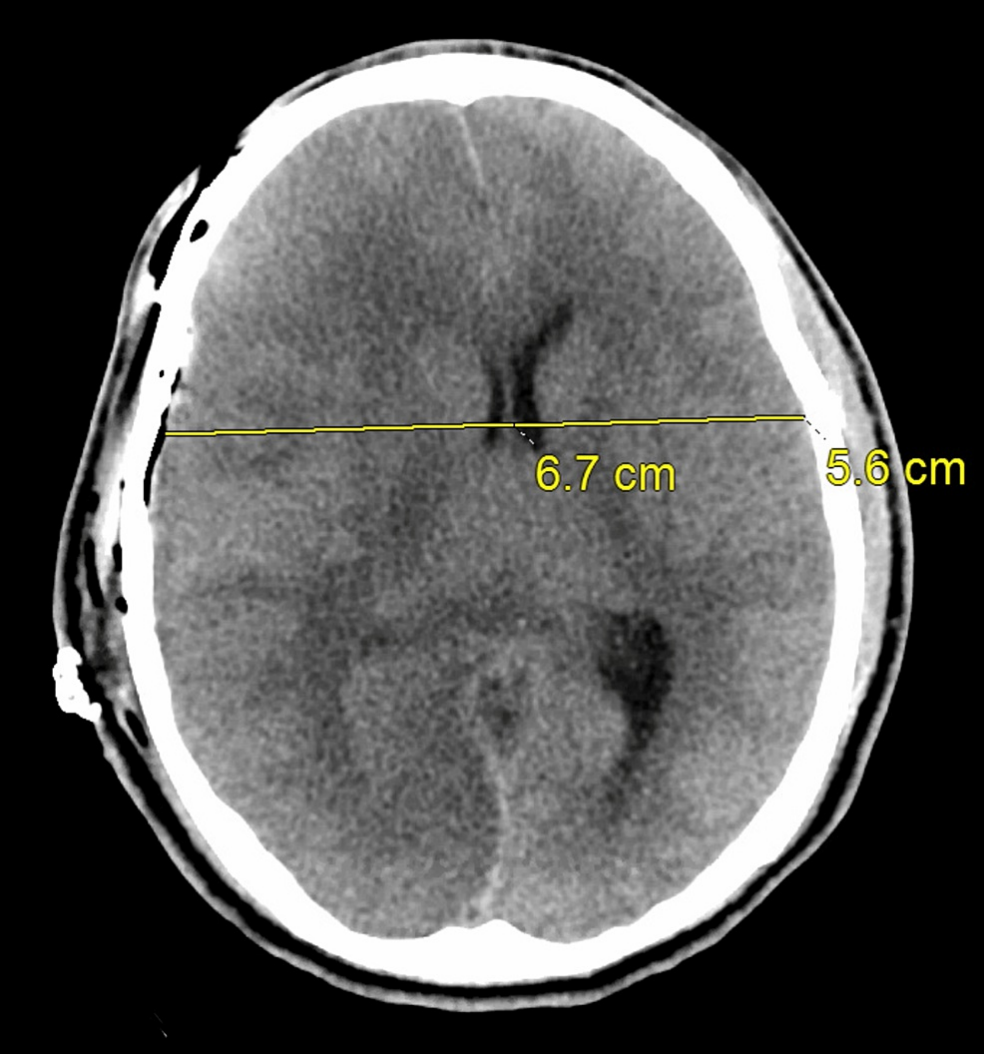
Head CT scan post decompressive craniectomy on day one shows developing ischemic changes involving the anterior right frontal convexity medial right occipital lobe extending into the posterior temporal lobe. CT: computed tomography
